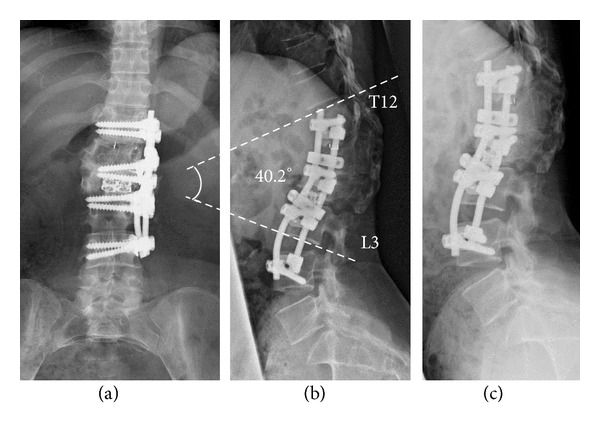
Postsurgical plain radiographs: (a) AP view showing the anterolateral fusion from T12 to L4 with interbody fusion between L2 and L3. (b) Lateral neutral view showing improved alignment measuring 40.2° from T12 to L3 and approximate 15° correction. (c) Lateral flexion view showing the stabilized lumbar spine.
